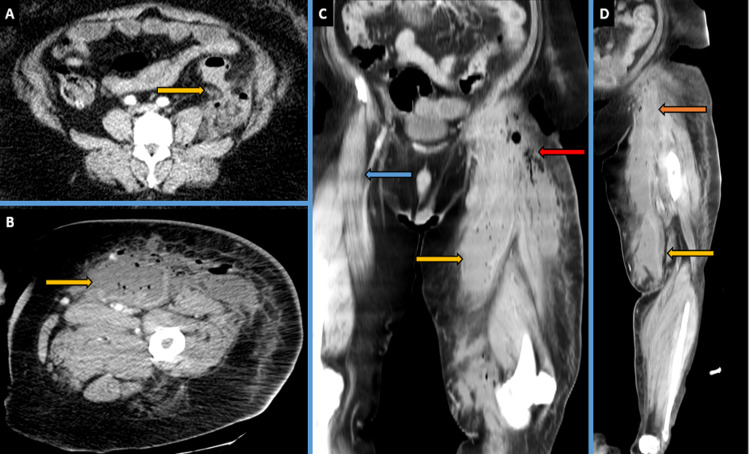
Pre-operative day one CT images (A) Axial contrast-enhanced computerised tomography (CECT) image showing left-sided colonic diverticulitis with surrounding inflammatory changes. (B) Axial CECT image of the left upper thigh showing rim enhancing abscess. (C) Coronal CECT image showing the supero-inferior extent of the left thigh abscess extending from the left inguinal region up to the left of the knee by yellow/red arrows, blue arrow depicting the normal side. (D) Oblique coronal CECT image showing the supero-inferior extent of the left thigh abscess extending from the left inguinal region up to the knee level.
